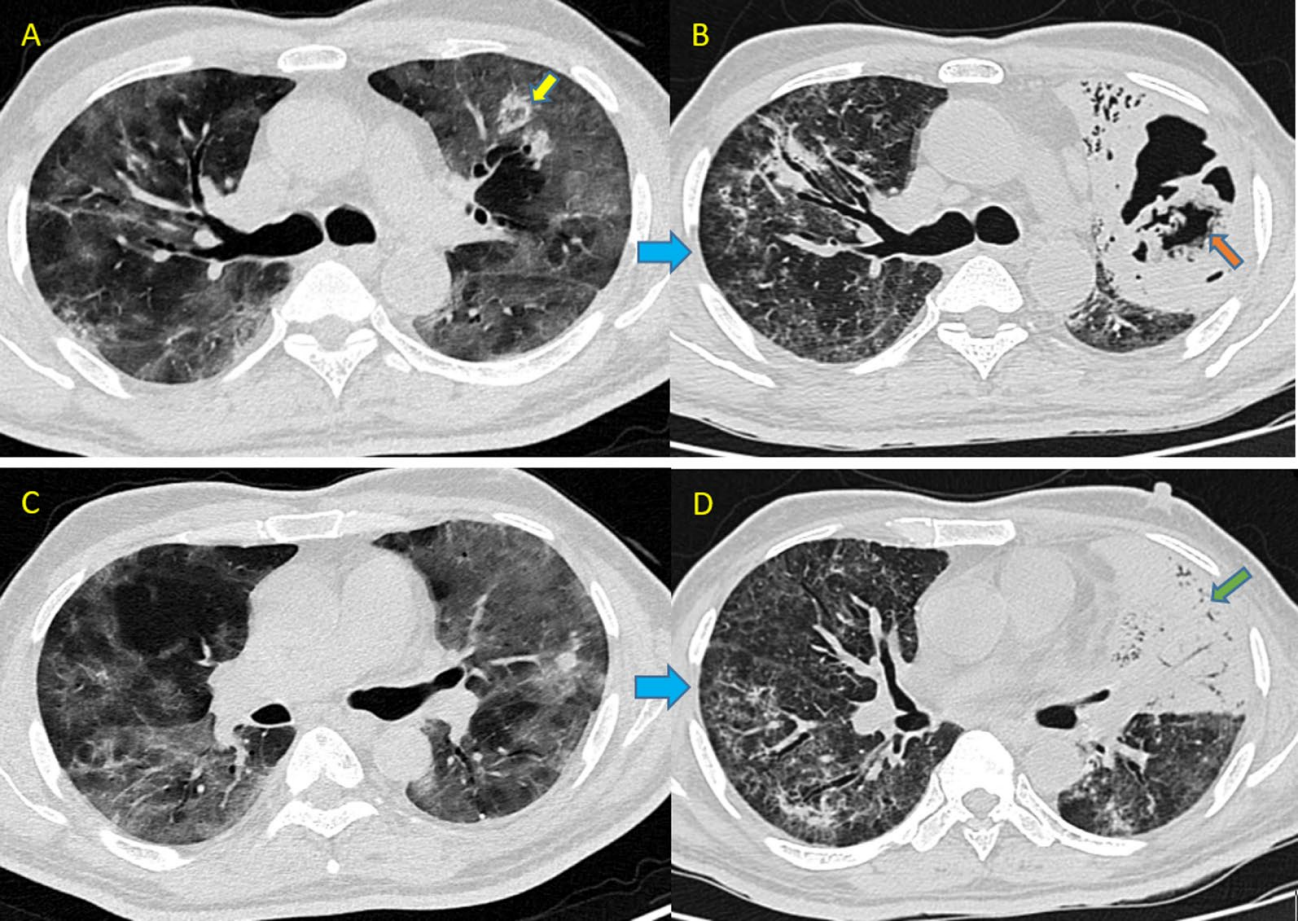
Imaging features of mucormycosis in Case 1 of COVID-19. A, B Axial HRCT scans show diffuse ground glass opacities in bilateral lung fields with few patches of reverse halo lesions (yellow arrow in A), without any evidence of cavitation. The scan was repeated after 21 days revealed cavitary lesions with soft tissue attenuating irregularly margined growth suggesting fungal infestation (orange arrow in B). C, D Revealed rapid progression of GGOs into consolidation (Green arrow in D)
